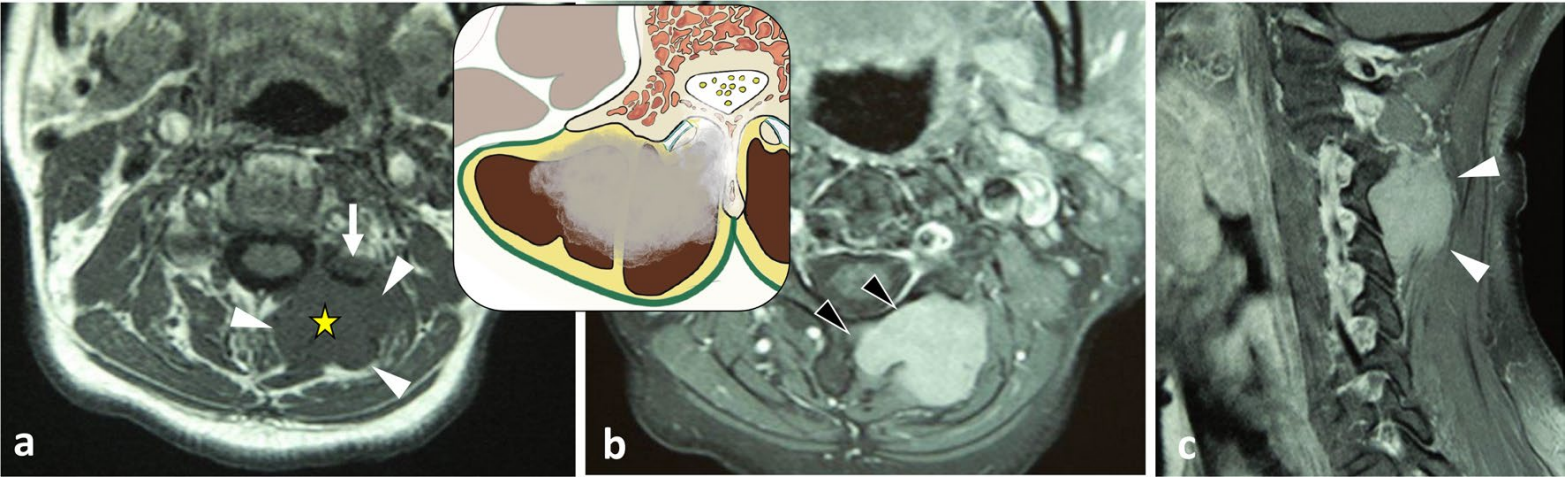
Leiomyoma* in a 60-year-old male with non-mechanical cervical pain. MRI scan shows a lobulated mass in left epaxial muscles (white arrowheads) with low signal intensity on T1WI (a) and enhancement on axial (b) and sagittal (c) contrast-enhanced fat-saturated T1WI. Several features are suggestive of vertebral bone invasion: disappearance of the fat plane separating the mass and the neural arch associated with a low signal of medullary bone of the articular process on T1WI (white arrow), disappearance of cortical bone and enhancement of medullary bone (black arrowheads) on contrast-enhanced T1WI. The yellow star shows the epicentre of the mass
